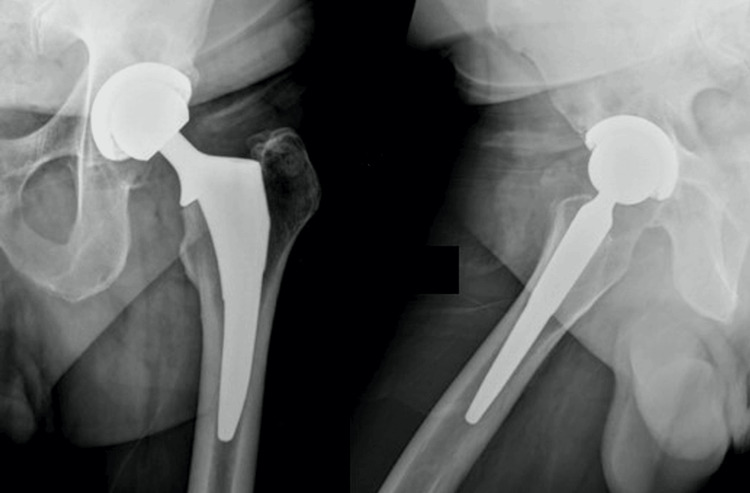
Anterior/posterior and lateral images of medial collared triple-tapered stem implanted via a direct anterior approach. X-ray images of medially collared triple-tapered (MCTT) stem at six weeks post-implantation.
